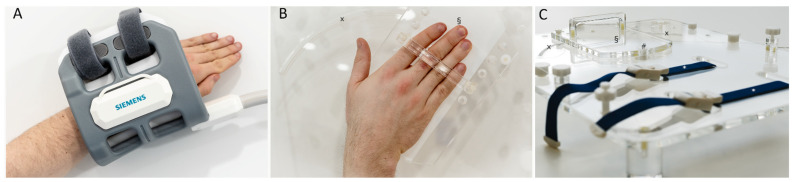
Coil and measurement setups during static reference and dynamic real-time MRI measurements. (A) For static measurements, a four-channel flex coil (Siemens Healthineers) was wrapped around the wrist to acquire the morphologic sequences. Shown are a left forearm and hand that were immobilized and imaged for reference purposes. (B) Positioning of a right wrist on the custom-made MRI-compatible movement device. The device has a mobile sliding tray (§) with a predefined range and trajectory of movement (x) onto which the hand was placed. (C) Tourniquets (*) were used to fix the forearm and prevent adaptive movement. The underside of the mobile sliding tray was coated with synthetic polytetrafluoroethylene (“Teflon”) to reduce friction. Centered underneath the wrist is the pivot point (#), which connects the mobile sliding tray and the fixed base plate. For dynamic measurements, a 24-channel spine matrix coil and an 18-channel body coil (both from Siemens Healthineers) were positioned underneath (spine matrix coil) and on top of the device (body coil) [not shown].
